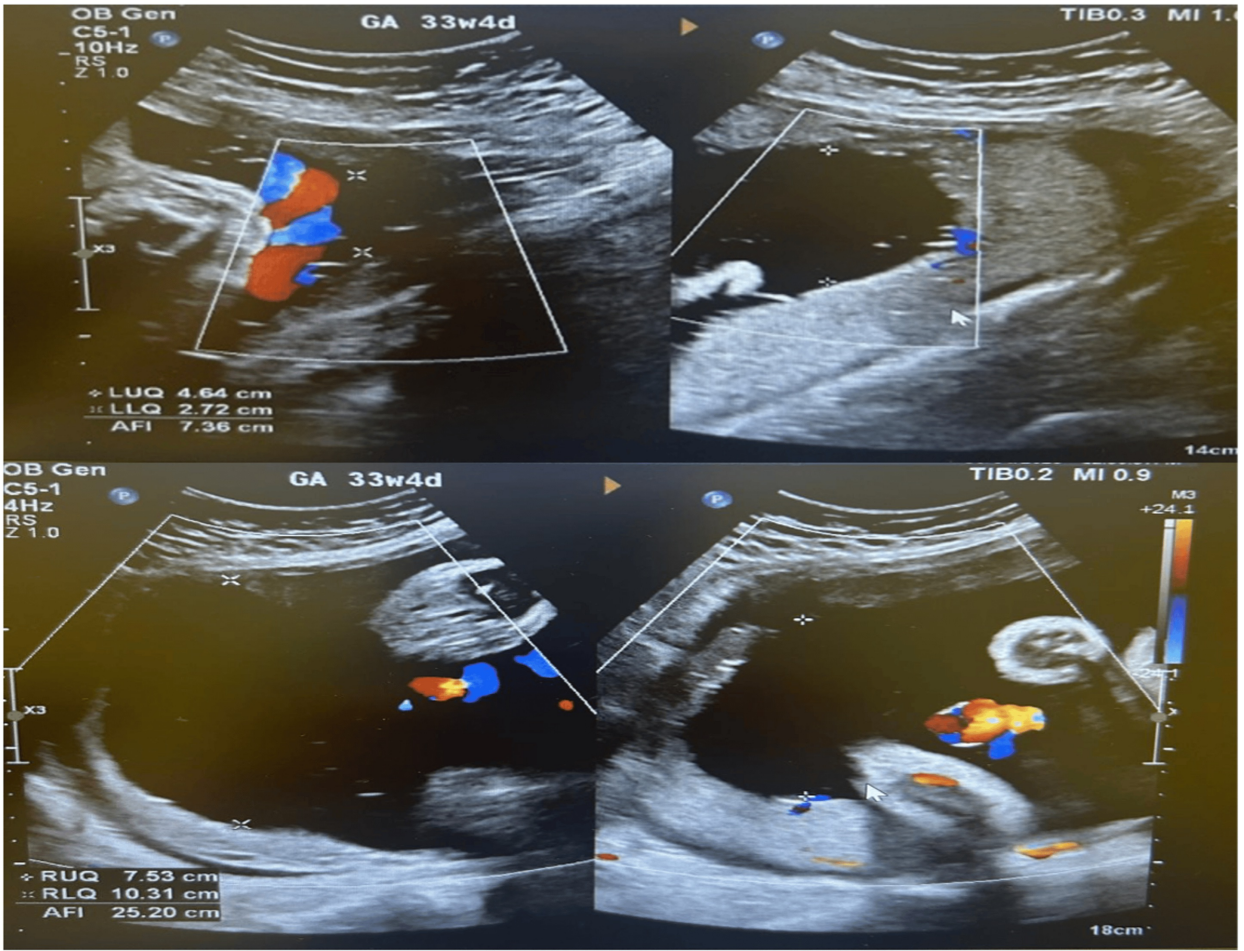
Ultrasound at 33+4 weeks demonstrating polyhydramnios (AFI: 25 cm) AFI: amniotic fluid index
